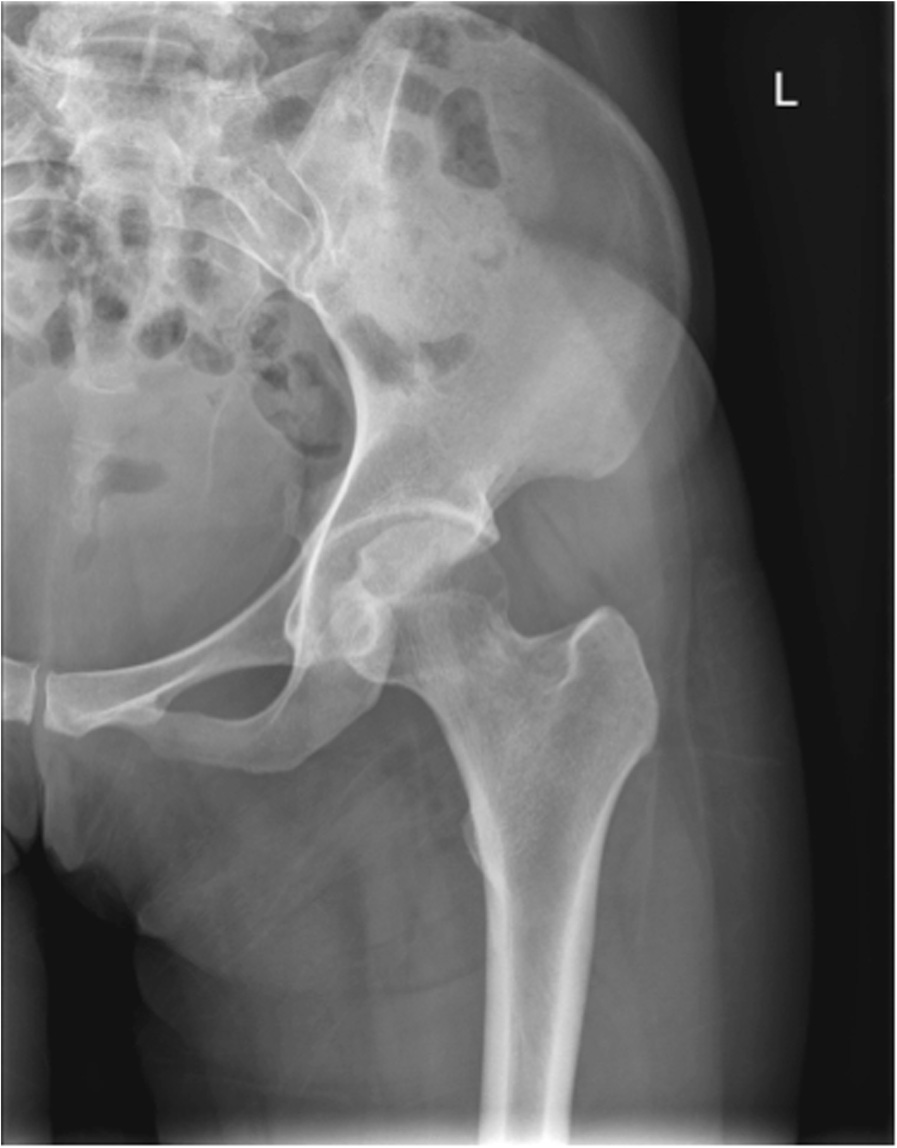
Normal X-ray left hip - Anteroposterior view.
